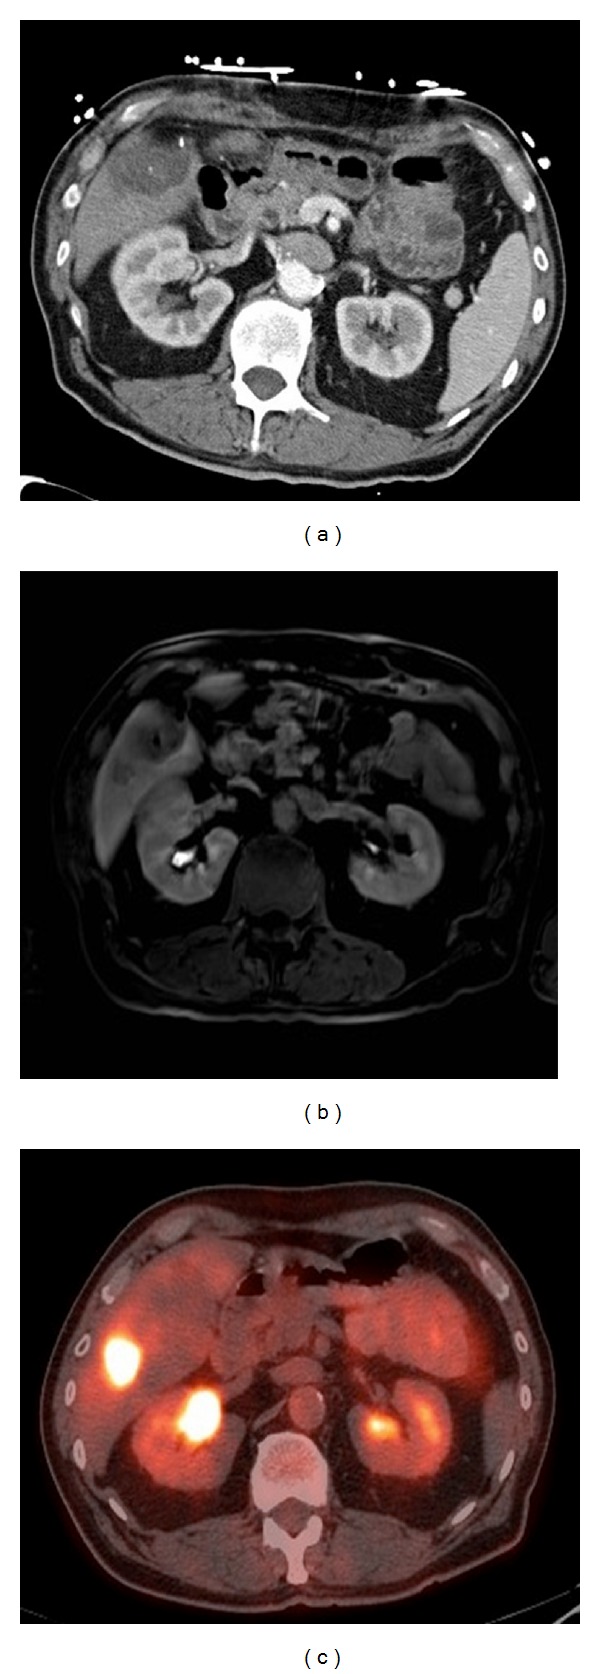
Liver metastases in the same patient compared with contrast-enhanced CT, Gd-EOB-DTPA-enhanced MRI and PET/CT scan. 76 y. male with history of rectal cancer after neoadjuvant chemotherapy and radiation followed by low anterior resection. Segment 5 and caudate lesions, now with segment 6 lesion not seen on CT scan (a) but present on PET (c) and MRI with Eovist (b).
